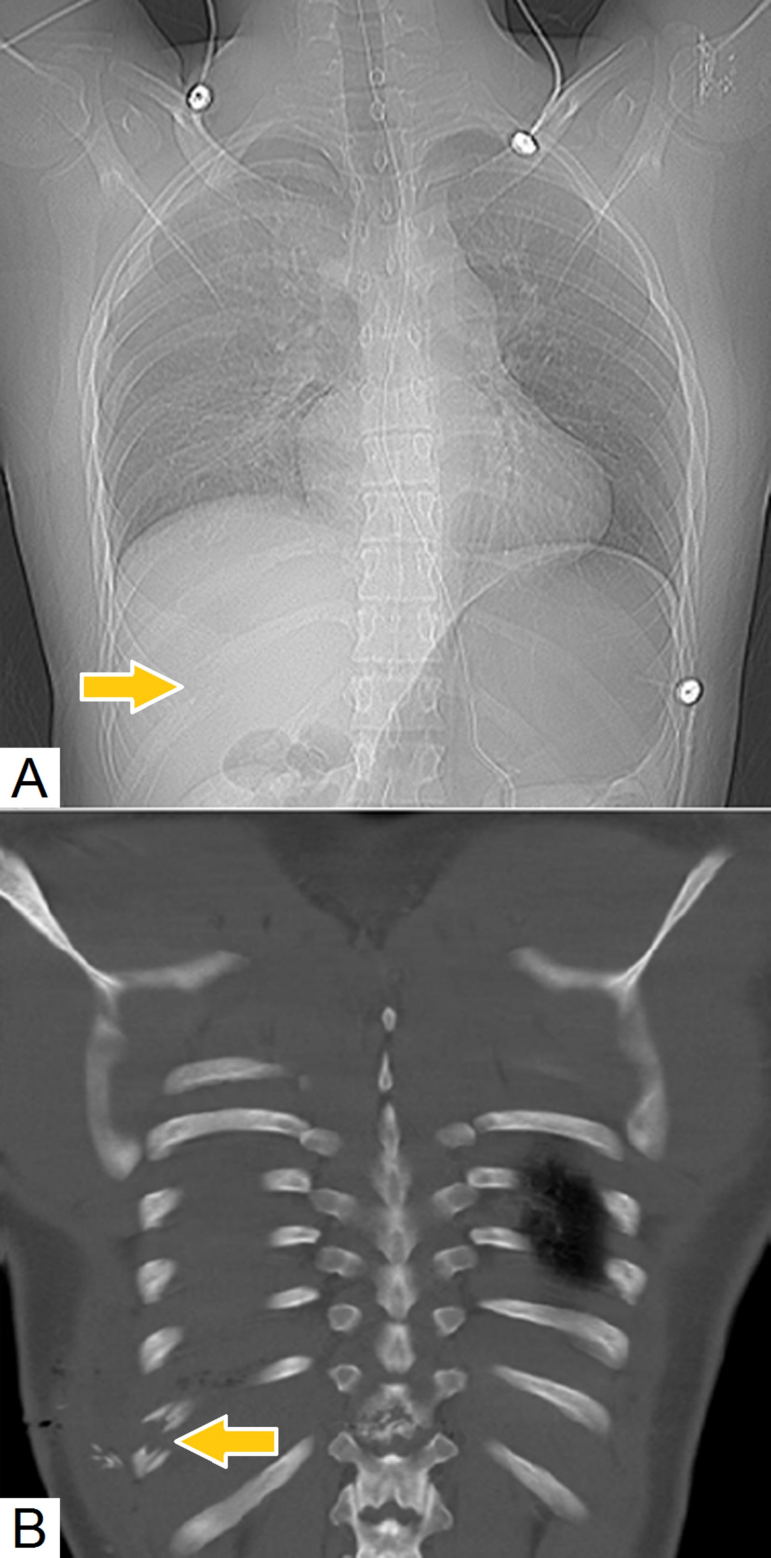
Depiction of rib fracture on scout film and thoracic computed tomography (A) Scout film of computed tomography (CT) depicting fracture of the right eleventh rib (arrow). (B) Thoracic CT in “bone” window settings confirm a fracture of the right eleventh rib (arrow).
